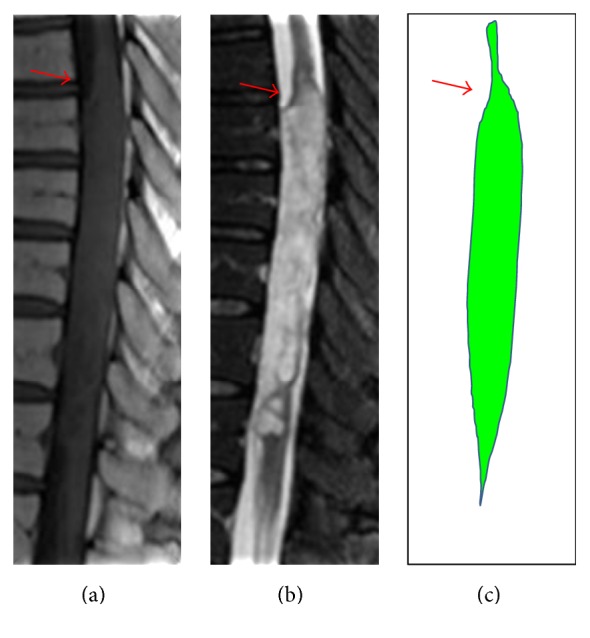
Bamboo leaf sign. The figure shows MRI of spinal subependymoma from our case. (a) T1WI. (b) T2WI. Sagittal MRI shows steep swelling of the spinal cord (arrows) and unusually large fusiform dilatation, resembling the shape of a bamboo leaf (c).
